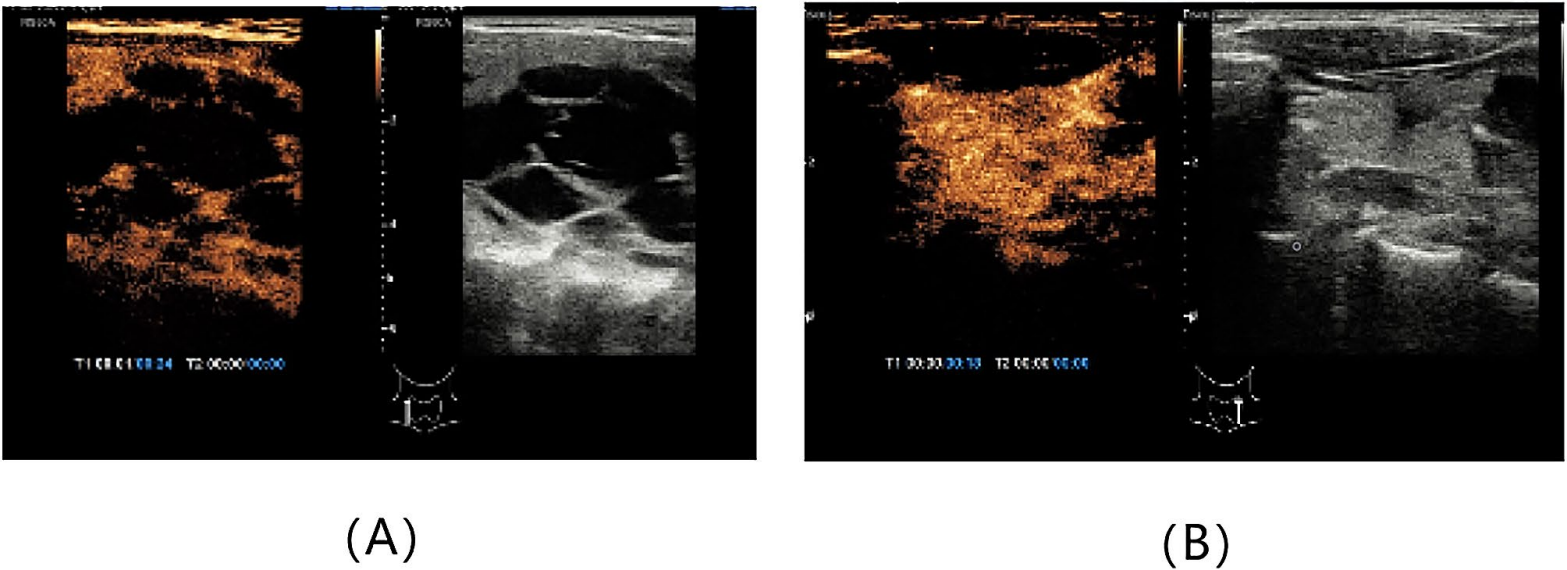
Thyroid contrast-enhanced ultrasound (CE-US) images. Multiple nodular goiter (A) and a parathyroid adenoma located in the middle and lower left thyroid lobe (B)
